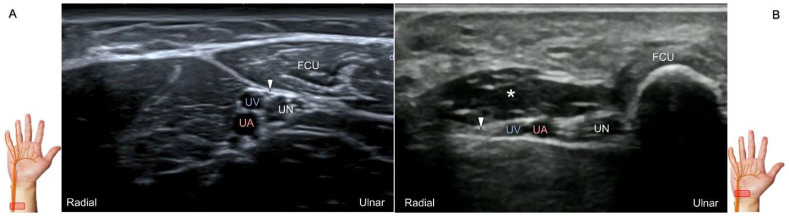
Sonographic imaging (short-axis view) of the palmar ulnar cutaneous nerve (A) and its entrapment in the presence of an accessory abductor digiti minimi muscle (B). Arrowhead: palmar ulnar cutaneous nerve; asterisk: accessory abductor digiti minimi muscle. UN: ulnar nerve; UA: ulnar artery; UV: ulnar vein; FCU: flexor carpi ulnaris tendon.
